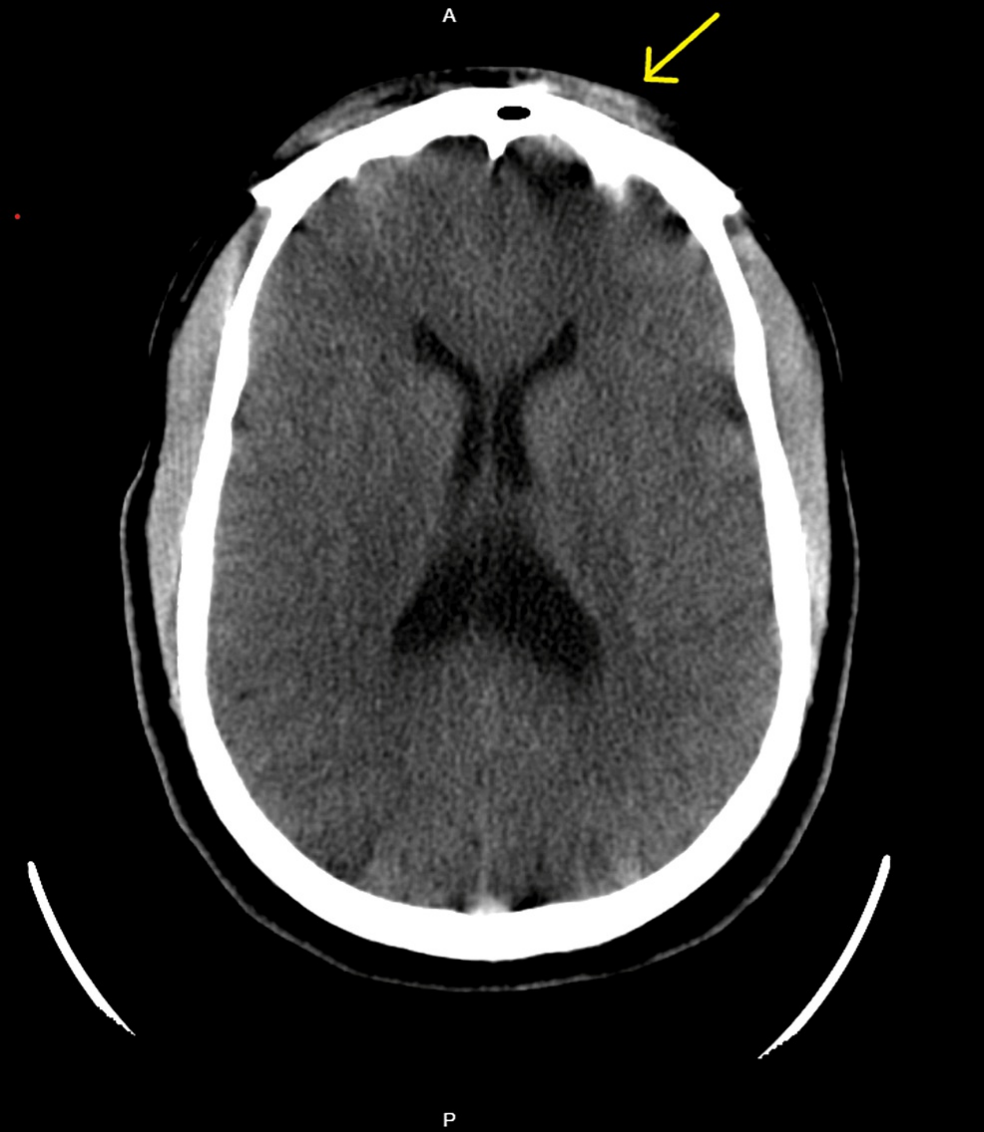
CT head shows a mild left frontal subcutaneous soft tissue swelling suggestive of a contusion or hematoma.
